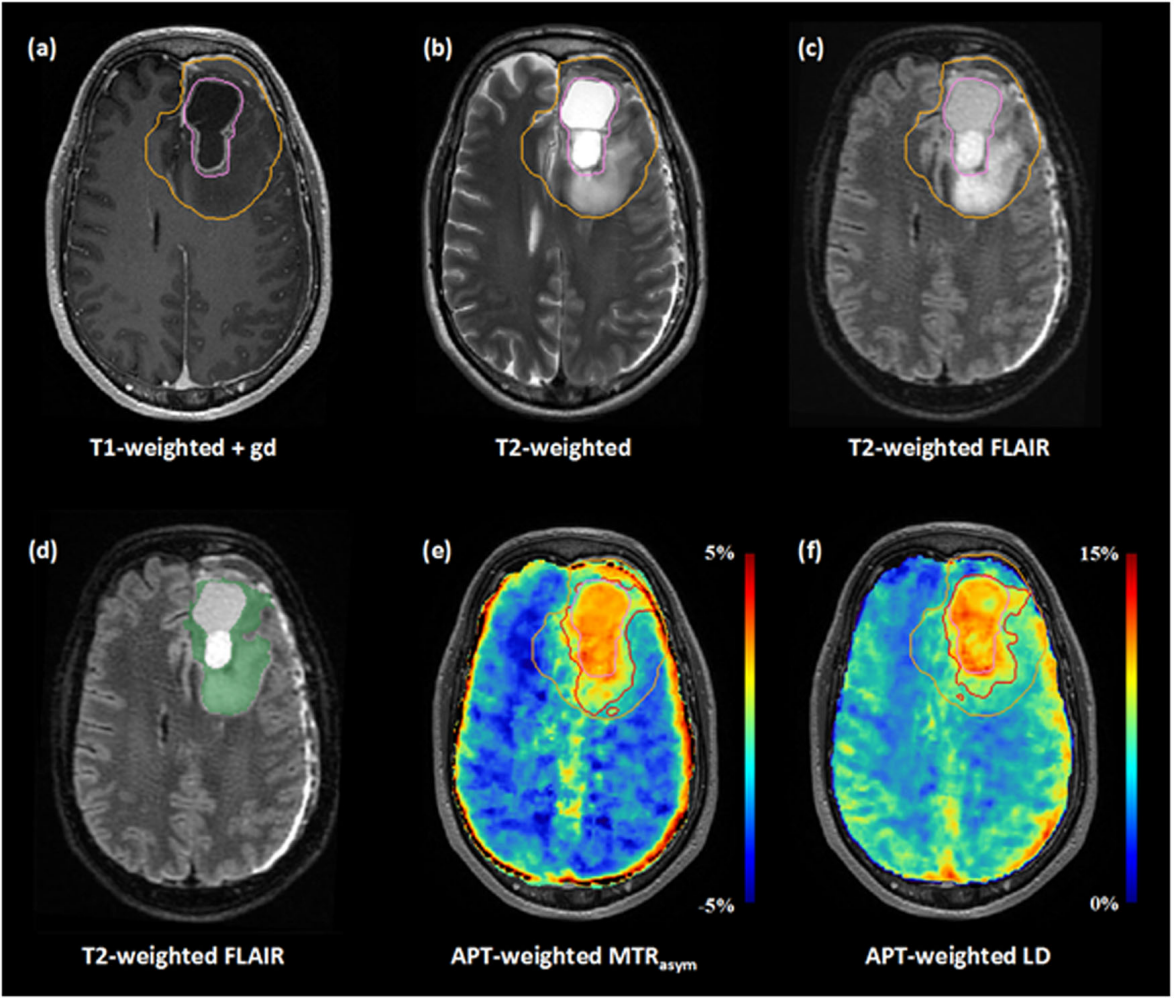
Target delineations of patient #2. The top row shows the conventional GTV (pink contour) and CTV (orange contour) on structural MRI (a–c). The automatic segmentation of residual contrast enhancement and peritumoral T2-weighted and T2-weighted FLAIR hyperintensities is shown as a green overlay on T2-weighted FLAIR MRI (d). Note that the resection cavity is not included in this segmentation. The conventional GTV (pink contour) and CTV (orange contour), as well as the GTV_bio,MTRasym_, and GTV_bio,LD_ (red contours), can be seen on the APT-weighted MTR_asym_ (e) and LD (f) map. Compared to the conventional GTV, the GTV_bio,MTRasym_, and GTV_bio,LD_ demonstrate an increase in volume of 81.2% and 52.4%, respectively. For abbreviations, see the “Abbreviations” section
